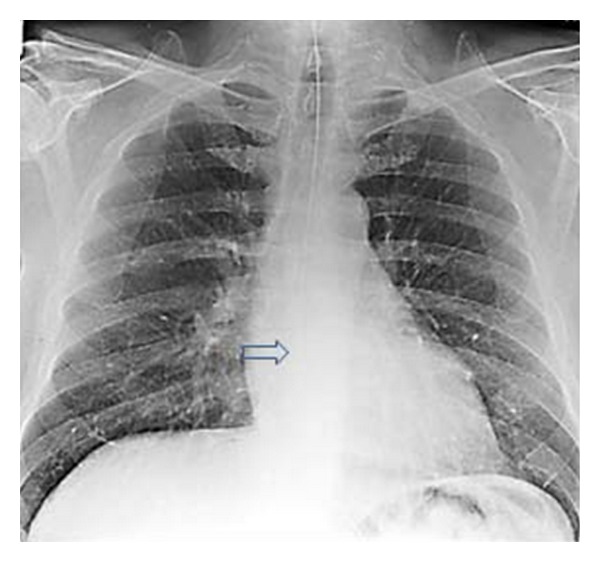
A CXR showing the tip of the NGT in the mid/lower esophagus. And it shows a portable chest radiograph on an intensive care patient that developed pleural effusions. A chest drain was placed to drain the left-sided pleural effusion. Bright red blood was obtained from the tube. A CT scan (Figure 28) obtained immediately revealed the tip of the catheter had entered the left ventricle. The patient was immediately taken for cardiac surgery and the tube removed without complications.
